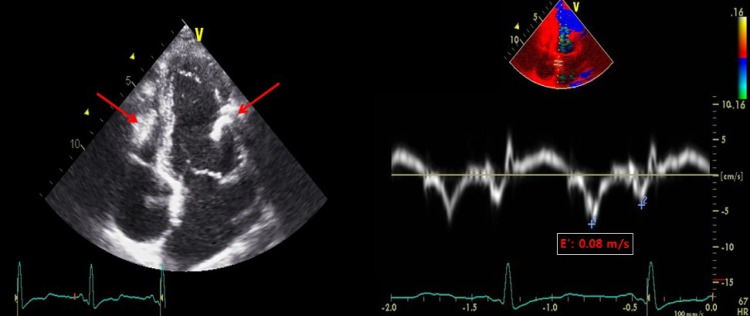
TTE showed multiple spindle-like extensive calcifications in myocardium (arrows). Tissue Doppler imaging revealed E’-velocity of the septal mitral annulus was 8 cm/s.
